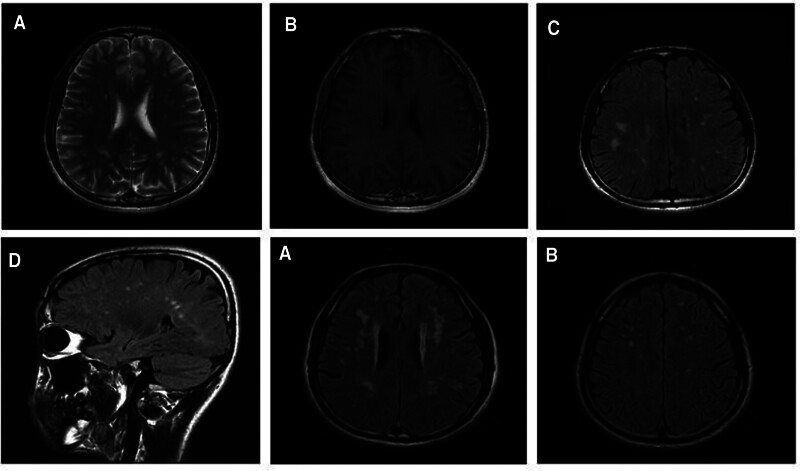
Brain MRI images of the patient (A–D) and his father a, b. Axial T2-weighted (A), T2 fluid-attenuated inversion recovery (C) and Sagittal T2 fluid-attenuated inversion recovery (D) images showed several discrete abnormal hyperintensity lesions in the periventricular deep white matter as well as frontal and parietal lobes of both cerebral hemi-spheres, most lesions were distributed perpendicular to the lateral ventricles, and some became confluent; T1-weighted contrast (B) images showed lesions were not enhanced. Axial T2 fluid-attenuated inversion recovery a, b. Images of the father showed more bilateral deep paraventricular white matter confluent lesions and more obvious brain atrophy. MRI = magnetic resonance imaging.
